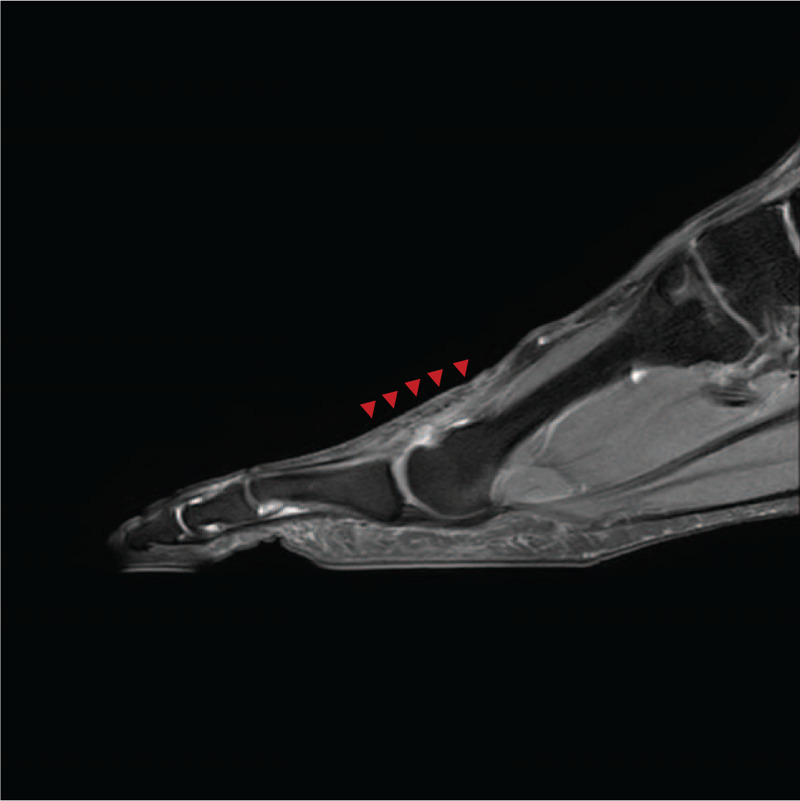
On the proton density-weighted sagittal magnetic resonance image taken 1 year after reconstruction, the second EDL tendon is well maintained. (red arrowheads).
